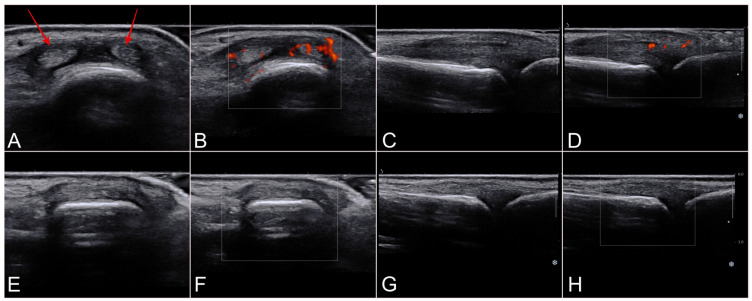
Preoperative high-resolution ultrasound (case 4, 24-year-old female). Transverse/axial ages in B-mode (A), color Doppler (B), sagittal images in B-mode (C) and color Doppler (D) showing hyperemia and (red arrows) gap through the EDM on the pathological side. Contralateral comparative images (E–H).
